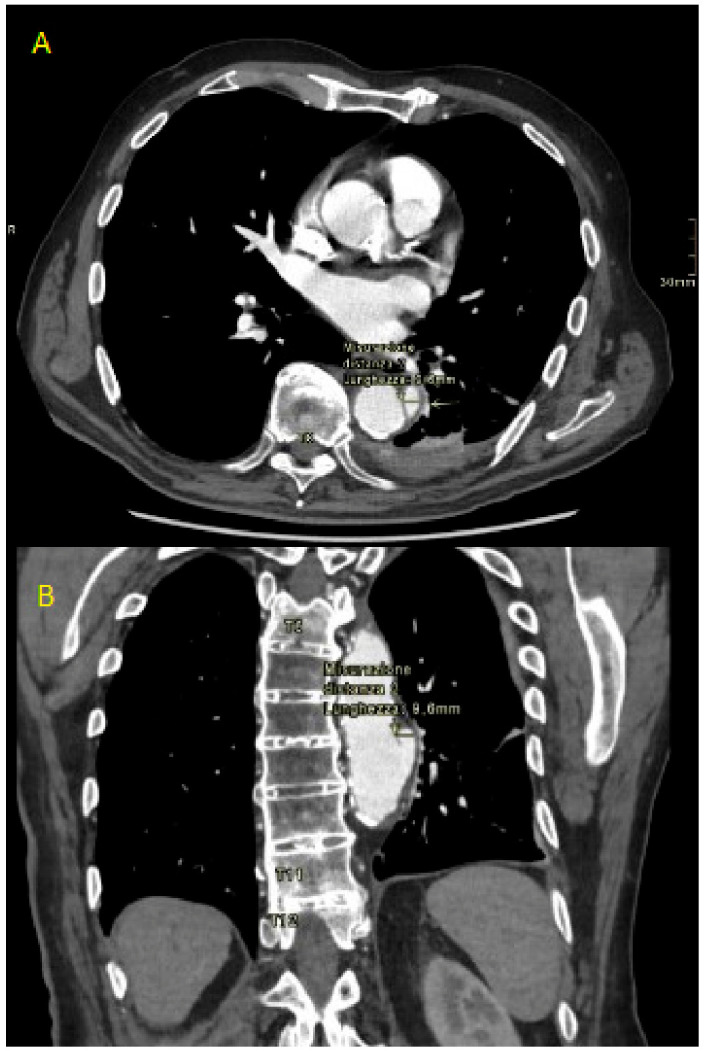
CT angiography ((A): axial; (B); coronal) demonstrating a penetrating aortic ulcer of the descending thoracic aorta associated with aneurysmal dilation and mural thrombus. The coexistence of ulceration and aneurysmal remodeling represents imaging markers of wall instability, highlighting the role of CTA in acute assessment.
